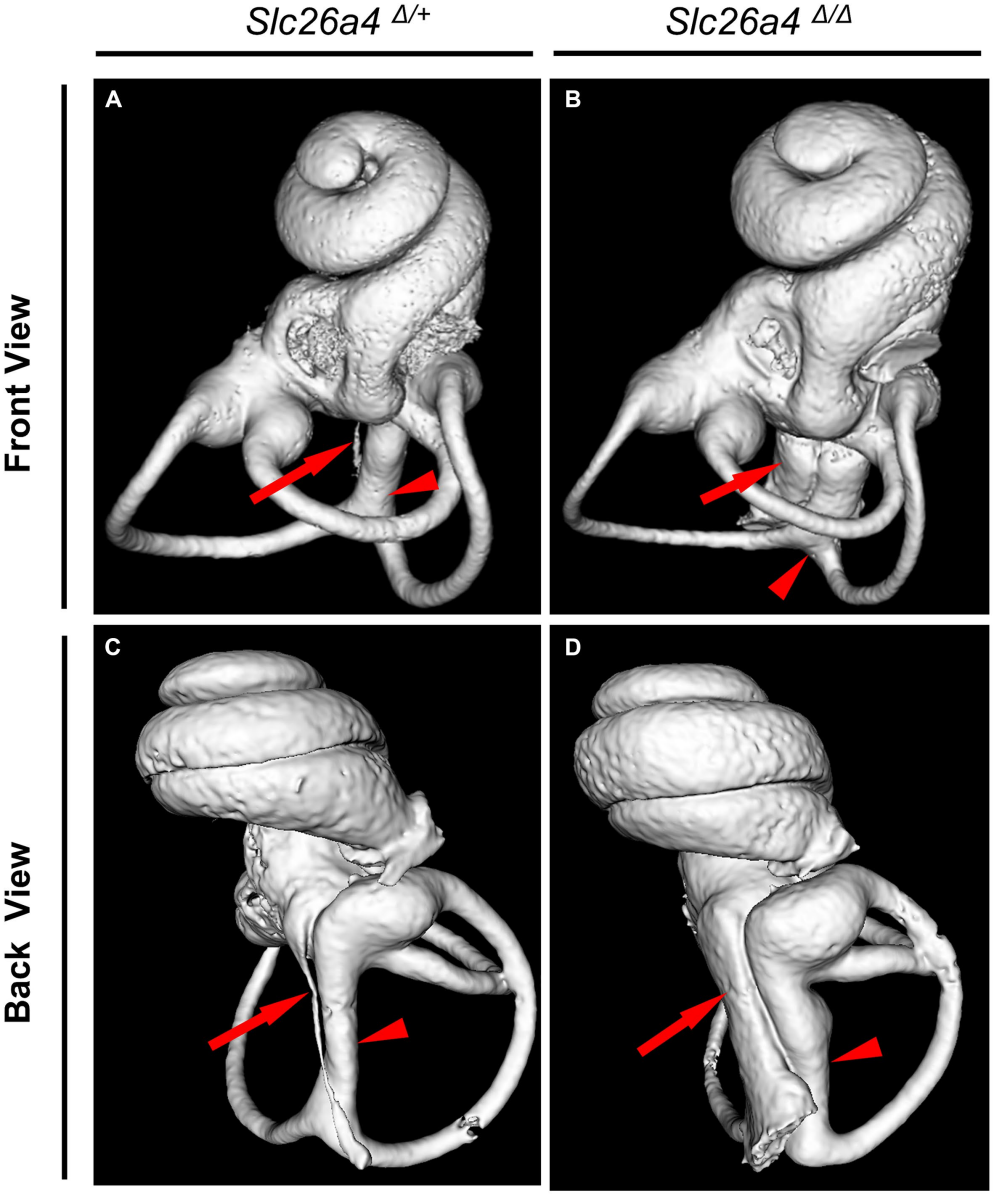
Bony labyrinth appearances. Panels (A,B) show the front views, and (C,D) show the back views of the bony labyrinth reconstructed by computed tomography. The bony labyrinths of common crus (arrowheads) and endolymphatic duct (arrows) are enlarged in the ear of *Slc26a4 ^Δ Δ^*
